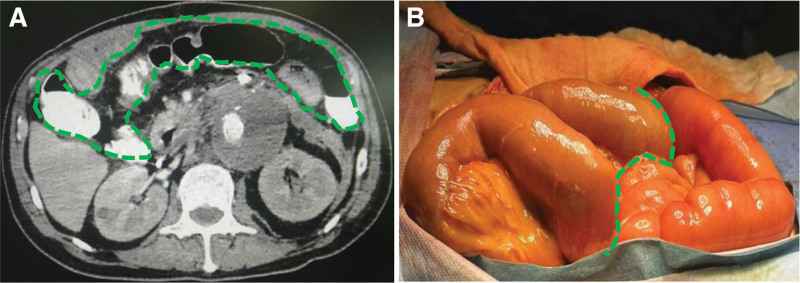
(A) Abdominal enhanced CT scan showing residual contrast in the colon, suggesting possible colonic ischemia. (B) Intraoperative findings showing extensive adhesion of the abdominal intestinal wall. The small intestine appears red and fresh on the right, while the colon is light gray, with no obvious signs of necrosis on the left.
